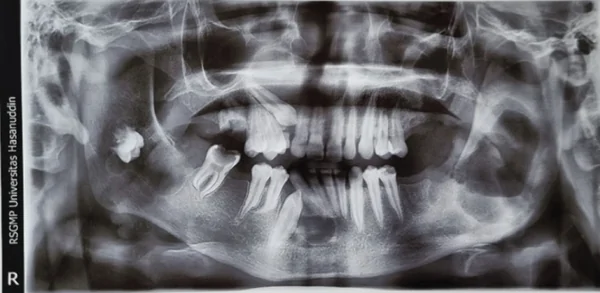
Postoperative panoramic radiographic control at 3 months.
